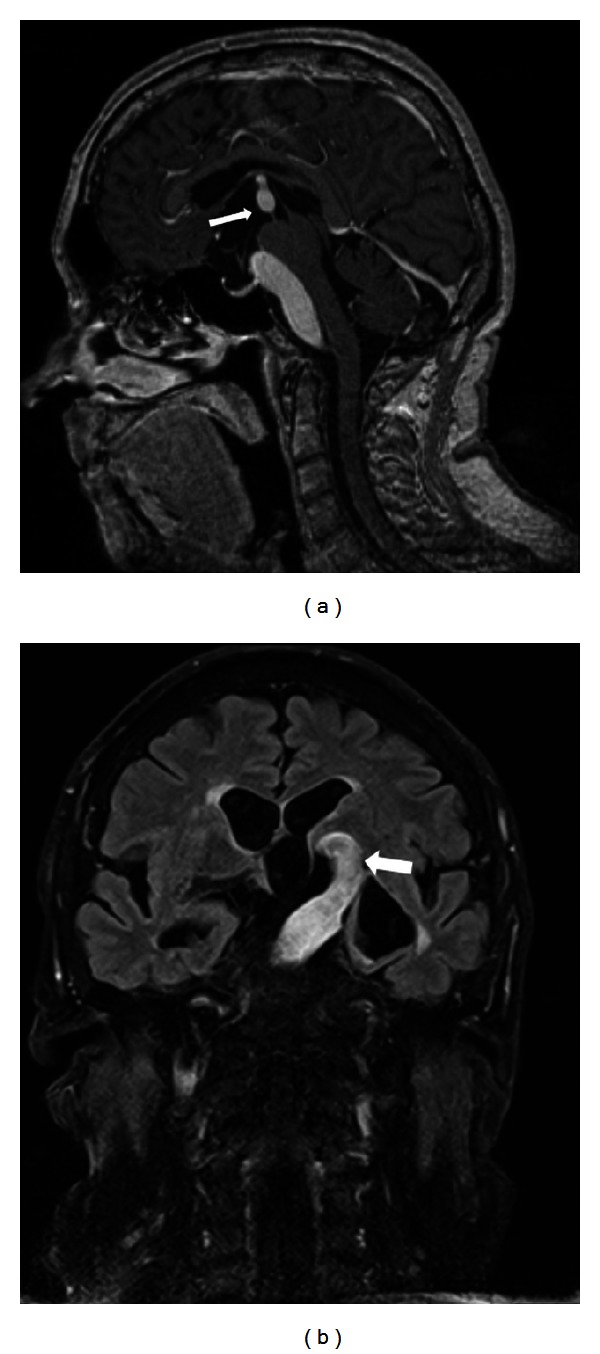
Magnetic resonance imaging (T1-weighted, contrast-enhanced). (a) Midline sagittal view (white arrow depicting dolichoectatic basilar artery elevating the floor of lateral ventricle). Note mass-effect on the brainstem. (b) Marked elevation of left optic tract (white arrow).
